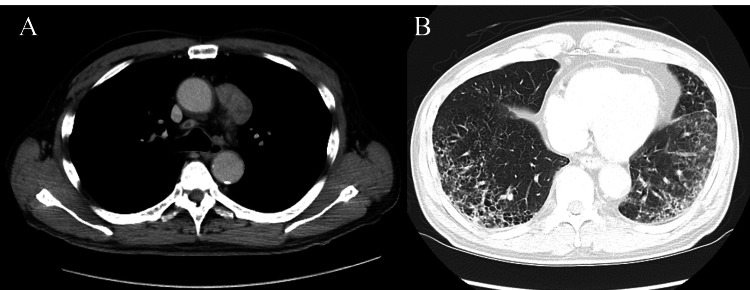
Computed tomography showing the first cancer. An anterior mediastinal tumor (A) alongside coexisting combined pulmonary fibrosis and emphysema (B) was revealed. The tumor, measuring 4.0 × 2.0 cm², appeared noninvasive.
